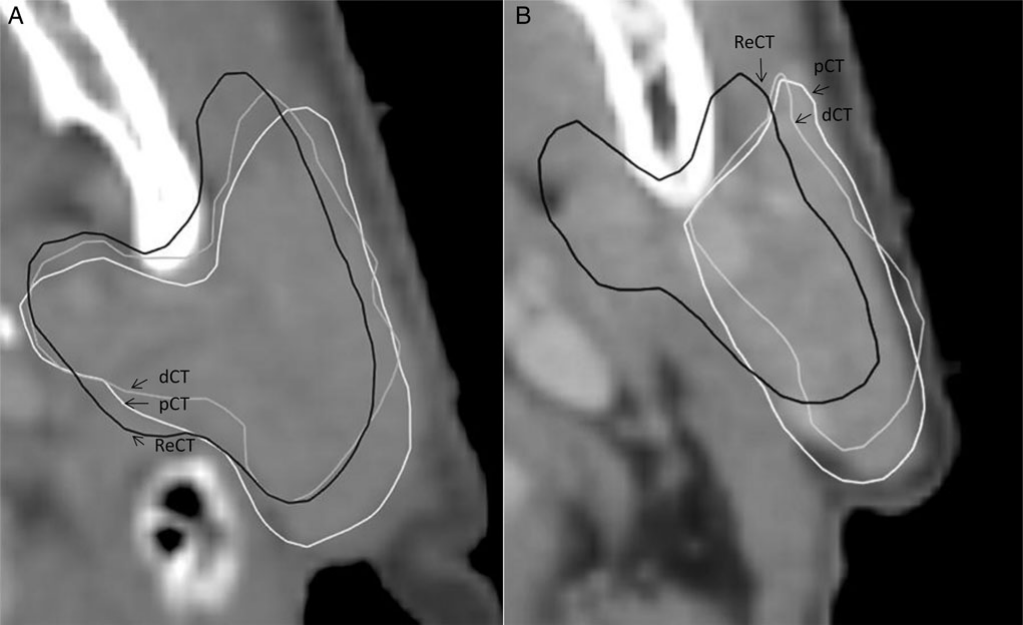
Visualization on the pCT of the patient with the lowest value of DSC for the parotid gland in two different CT slices. In some slices (A) the structures are similar, whereas large differences are observed between the dCT and the ReCT structures in other slices (B). The dCT structure resembles the pCT structure, and the large difference between the parotid on the pCT/dCT and the ReCT can, in part, be explained by intraobserver variations in the delineation.
